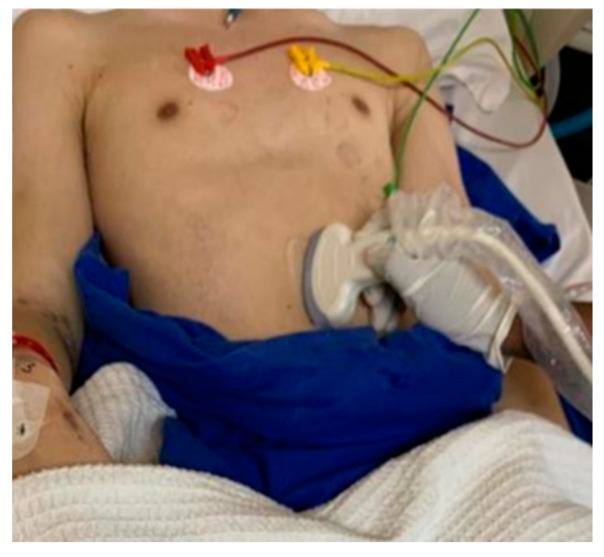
Placement of the ultrasound probe at the subxiphoid point.
